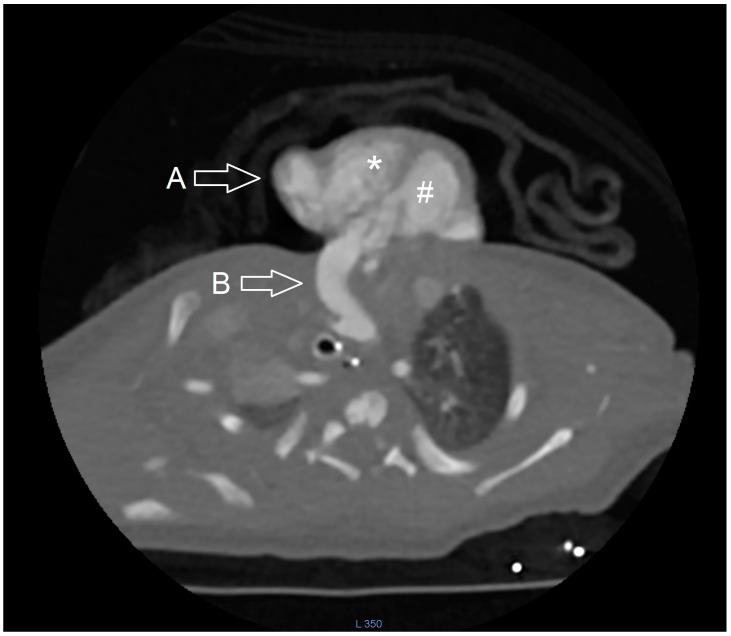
Axial chest computed tomography angiography (CCTA) scan showed a complete protrusion of the heart without the pericardium through the anterior chest wall. Arrow A—two-chamber long axis view of ectopia cordis. Arrow B—The aorta is positioned directly over the ventricular septal defect, passing into the right-sided aortic arch and then the descending aorta, which passes to the mediastinum. *—right ventricle, #—left ventricle.
